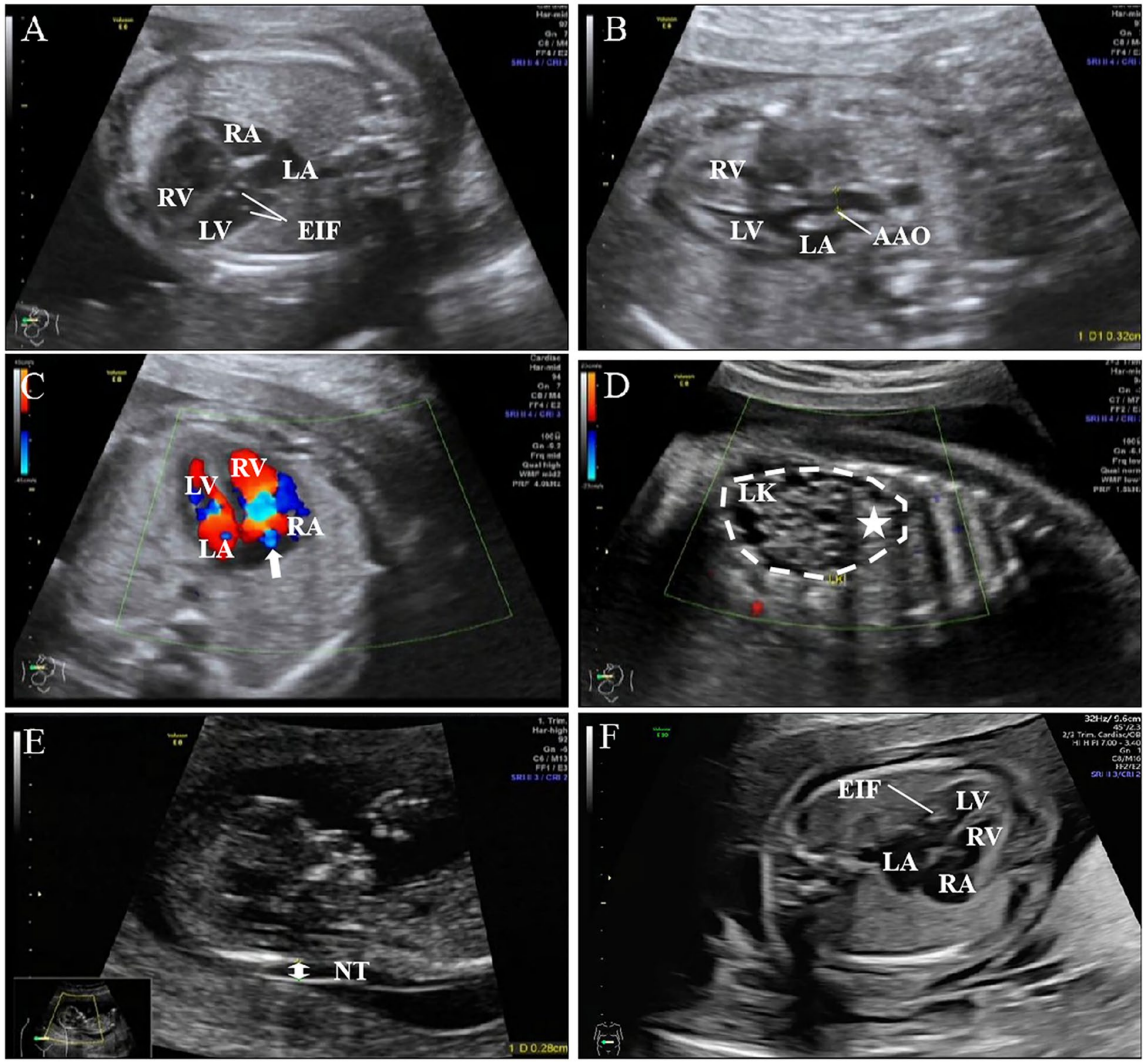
Ultrasound findings in two WS foetuses. Foetus 1 ultrasound showed (A) a left ventricle diastolic transverse diameter of 0.66cm, a right ventricle diastolic transverse diameter of 1.06cm, the left ventricle was significantly smaller than it is shown, the left ventricle was seen in the left ventricle of two echogenic solid points, the diameter about 0.13cm, 0.15cm, (B) the ascending aorta internal diameter of 0.32cm, the ascending aorta internal diameter narrowing; (C) a small amount of tricuspid regurgitation (the white arrow); (D) the left side of the polycystic dysplasia kidney (as indicated by a white star). (E) No significant cardiovascular abnormalities were seen in foetus 2; only a thickening of the NT of 2.8 mm was suggestive (double-sided arrow), and (F) an echogenic spot was seen in the left ventricle of foetus 2. Abbreviation: LA: left atrium; LV: left ventricle; RA: right atrium; RV: right ventricle; EIF: Echogenic intracardiac focus; AAO: Aorta ascendens; LK: Left kidney; NT: Nuchal translucency
